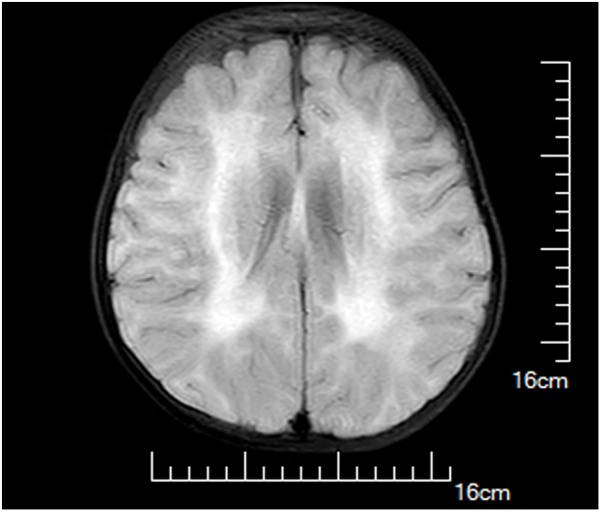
Brain magnetic resonance images of the proband at 1 month of age. Hyperintensity on T2-weighted images, T1-weighted images in parietal and occipital lobes, and diffusion-weighted image (DWI) as well as hypointensity on fluid attenuated inversion recovery (FLAIR) image were observed.
